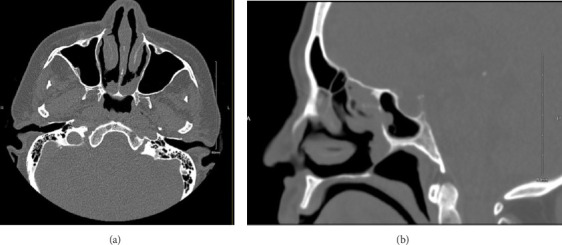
(a) Axial noncontrast CT scan of the paranasal sinuses (bone window) showing bilateral choanal atresia. (b) Sagittal noncontrast CT scan of the paranasal sinuses (bone window) showing choanal atresia.
